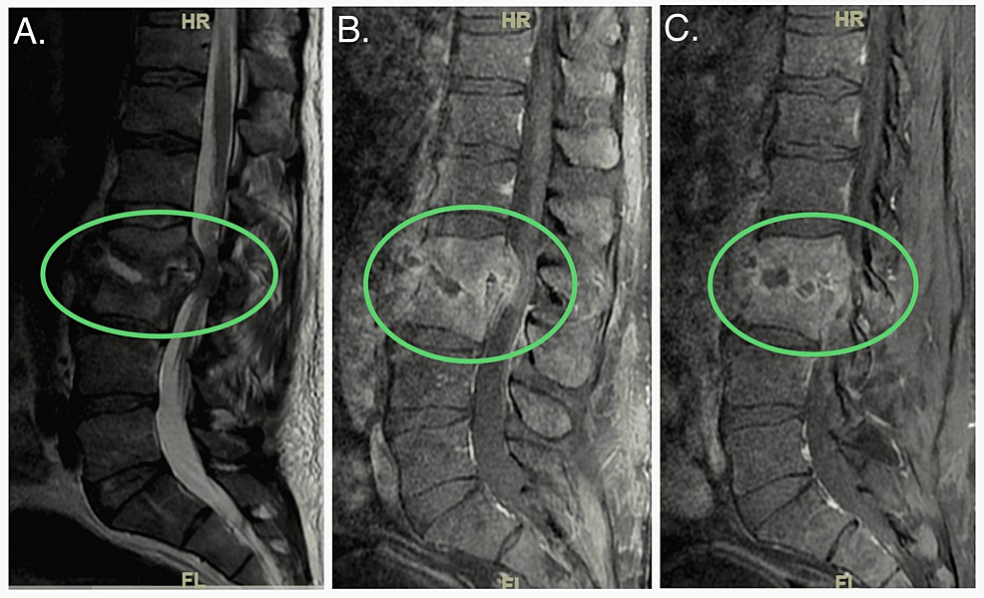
Multi-planar images from the initial MRI showing L2 and L3 discitis and osteomyelitis with the extension of infection into adjacent paraspinal soft tissues and the epidural space with marked compression of the thecal sac and nerve roots (green circles). MRI: magnetic resonance imaging.
